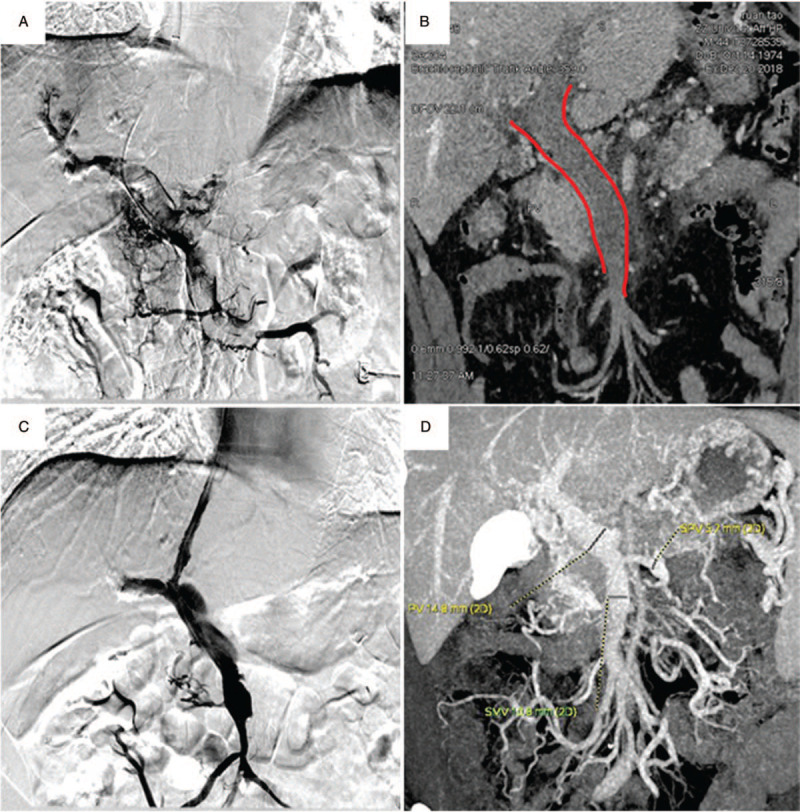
Establishing a shunt combined thrombectomy device via the TIPSS route. A 52-year-old man hospitalized with portal hypertension and diagnosed with protein S insufficiency (A) Acute thrombosis portal vein, splenic vein and superior mesenteric vein – diagnostic contrast agent injected into the abdominal vein; (B) Preoperative enhanced CT showed extensive portal vein thrombosis, Red line; (C) Controlled angiography during lysis- patency of superior mesenteric vein and main portal vein; (D) Enhanced CT angiography revealed superior mesenteric vein and portal vein trunk without thrombus attachment.
